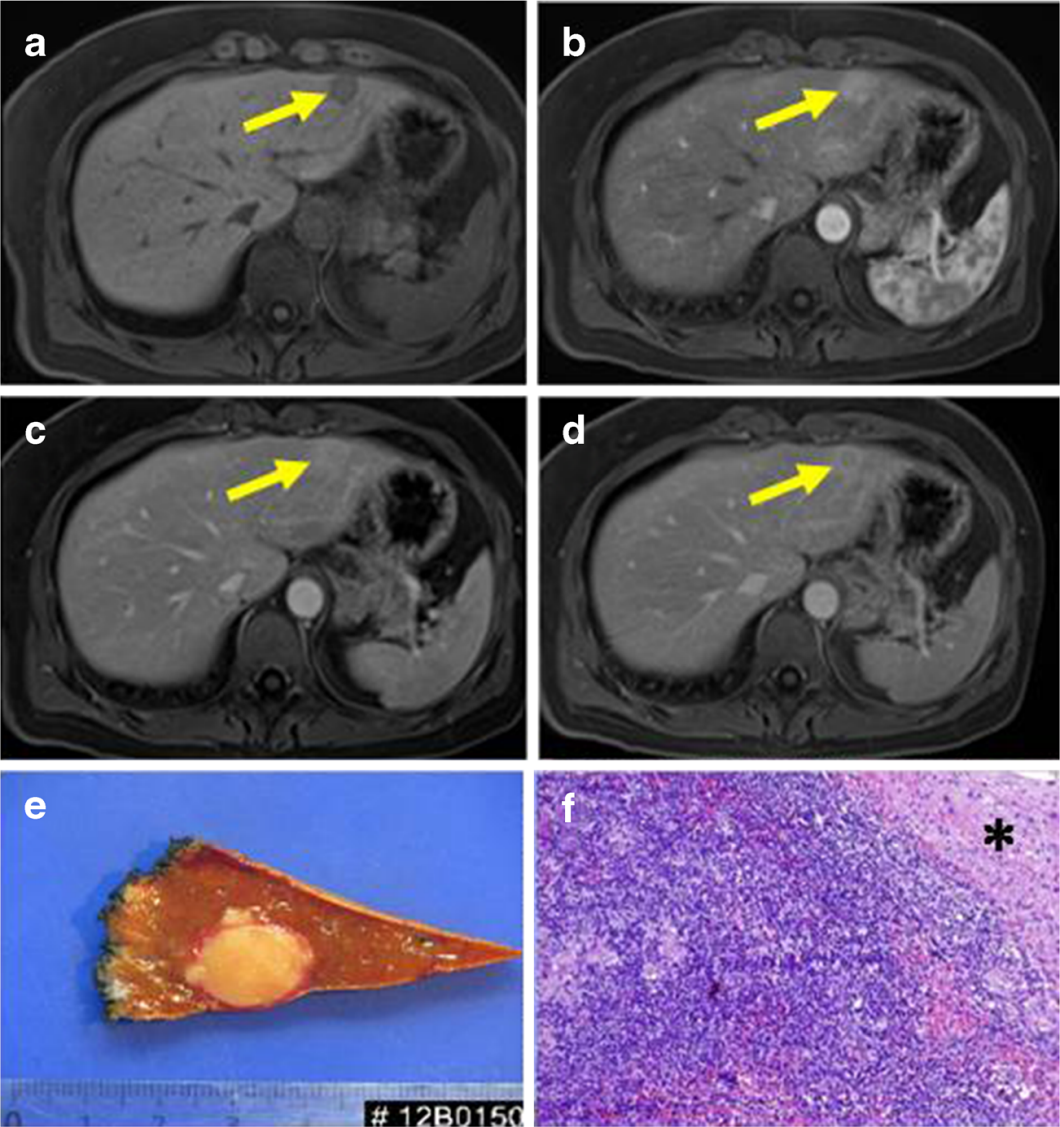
PATHOLOGY: IMT of the liver. Axial reformations of T1-weighted MR before and after intravenous gadolinium injection. Well-defined, hypointense lesion on T1-weighted images (a) which presented weak enhancement in the arterial phase (b) and wash-out on portal and equilibrium phases (c-, d) located in hepatic left lobe. Capsule was present. e Macroscopic view of partial hepatectomy obtained after surgical excision of the lesion. A solid, well-defined and yellowing appearance mass is showed. f The lesion demostrated an expansive chronic inflammatory infiltrate of plasmatic cells, lymphocytes and macrophages. The nearby hepatocytes (*) were normal
